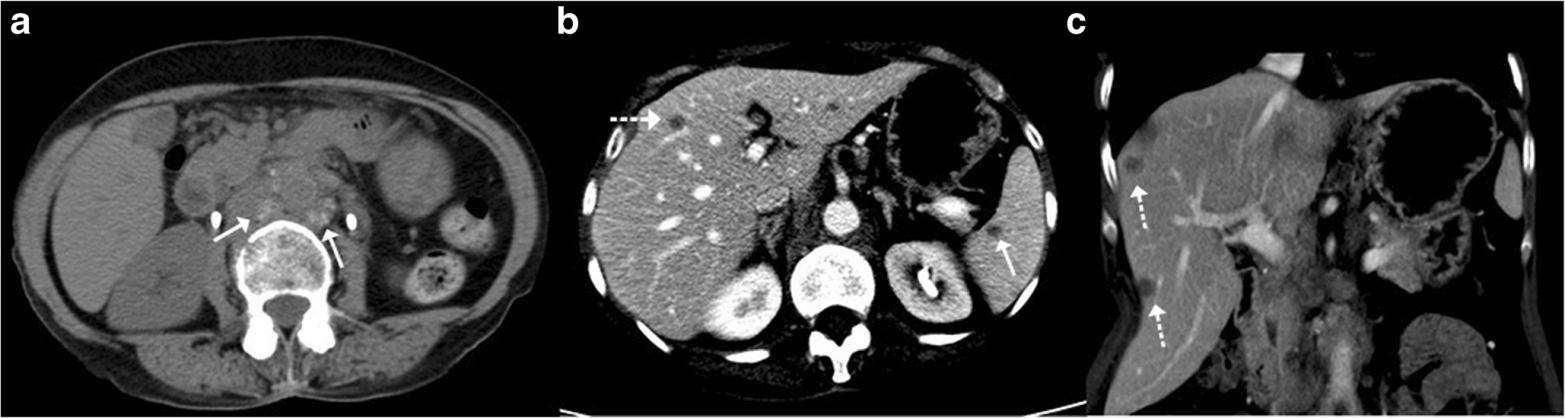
a Unenhanced CT of a 65-year-old woman with a history of weight loss showing retroperitoneal nodes with calcification (*arrows*). b, c Post-contrast CT images showing small hypodense lesions in both lobes of the liver (*dashed arrows*). Biopsy from the retroperitoneal nodes revealed metastases from mucinous adenocarcinoma
